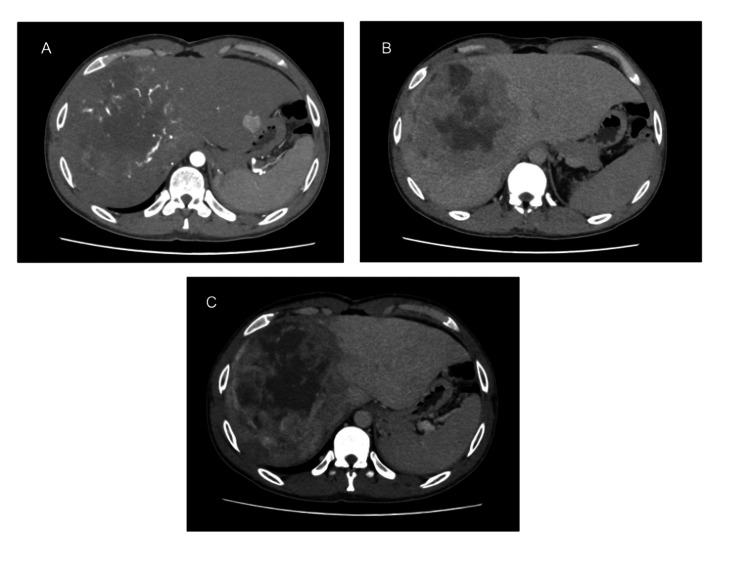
Triple phase axial CT images Triple phase axial CT images (A—arterial phase, B—delayed phase, C—venous phase) demonstrating a large hepatic mass in segment VIII with arterial phase enhancement, central necrosis, abnormally dilated feeding arteries, early venous washout, and delayed peripheral enhancement. A second lesion is seen in segment II of the left hepatic lobe, measuring 2.2 × 1.9 cm, with arterial enhancement and subtle delayed washout.
